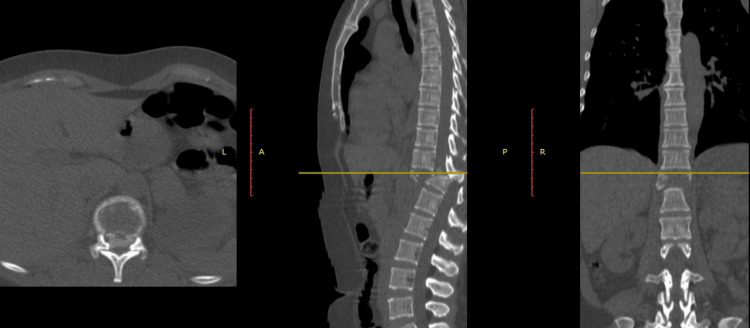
Case 5: initial CT scan with axial, coronal, and sagittal views of the injury, showing anterior displacement of the T11 vertebral body over T12.
